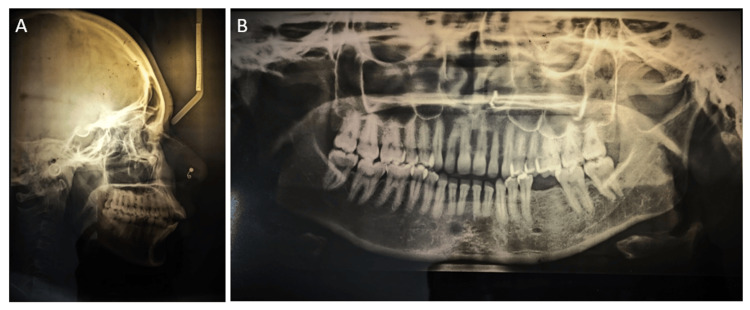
Pretreatment radiographs. (A) Lateral cephalogram, (B) orthopantomogram
